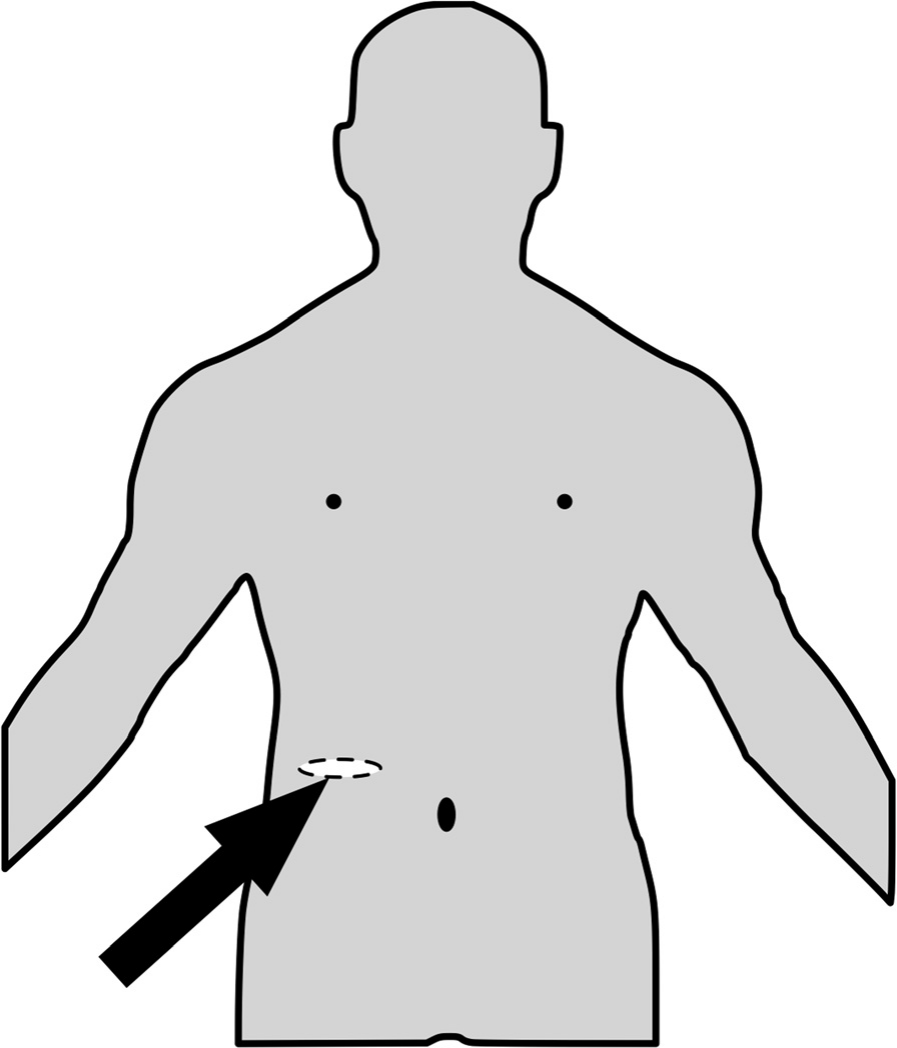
Transducer location. Location of the ultrasound measurement (dashed region). The transducer was positioned horizontally between the lowest rib and the anterior iliac crest
